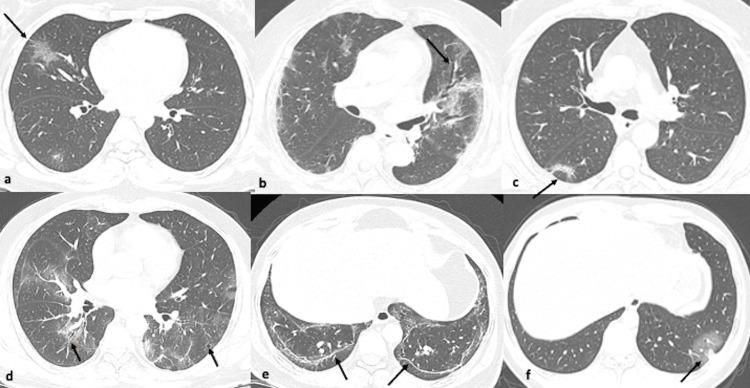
(a-f) Axial CT images from different known COVID-19 patients showing pattern of findings (arrows). Ground glass with crazy paving (a); ground glass with bronchial dilatation (b); reverse halo sign resembling organizing pneumonia (c), small cysts and bronchial dilatation (d), lower lobes parenchymal bands (e) and vascular dilatation and target/halo sign (f).
